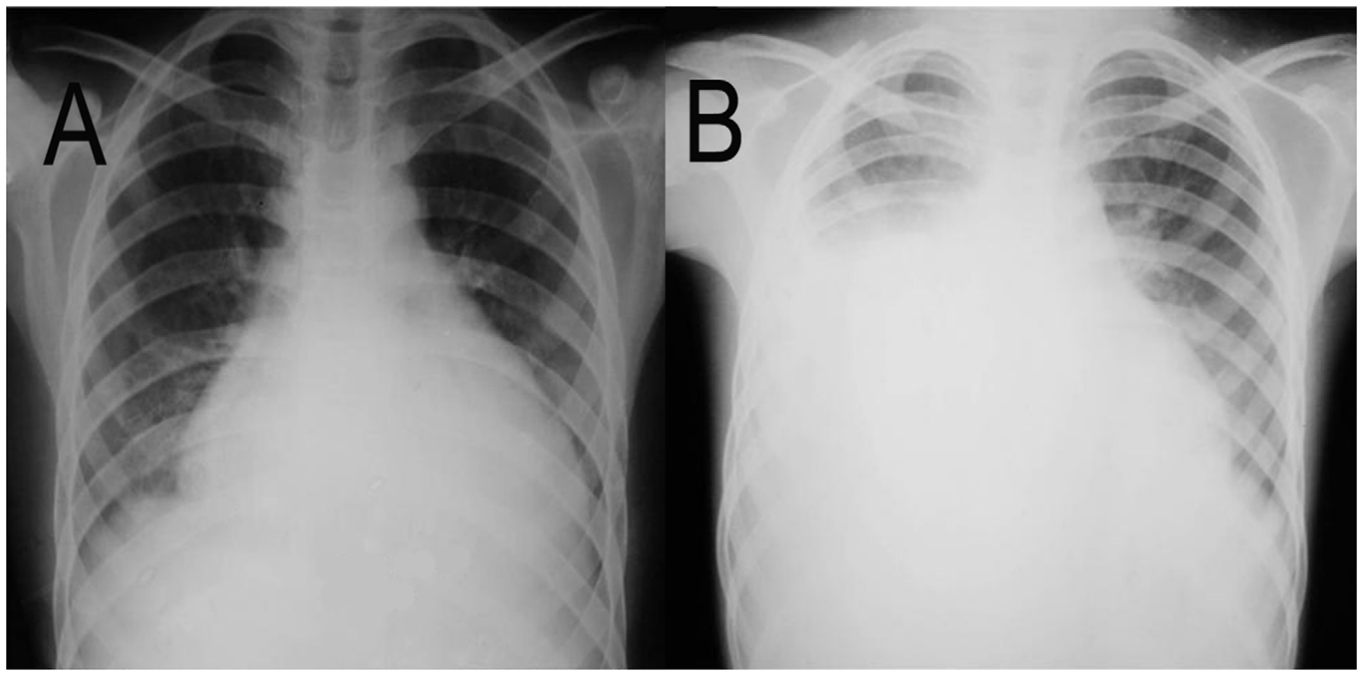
Chest radiographs showing cardiac enlargement and pulmonary congestion in acute Chagas disease. Patient # 6 (A), 16 years old; patient # 7 (B), 09 years old.
